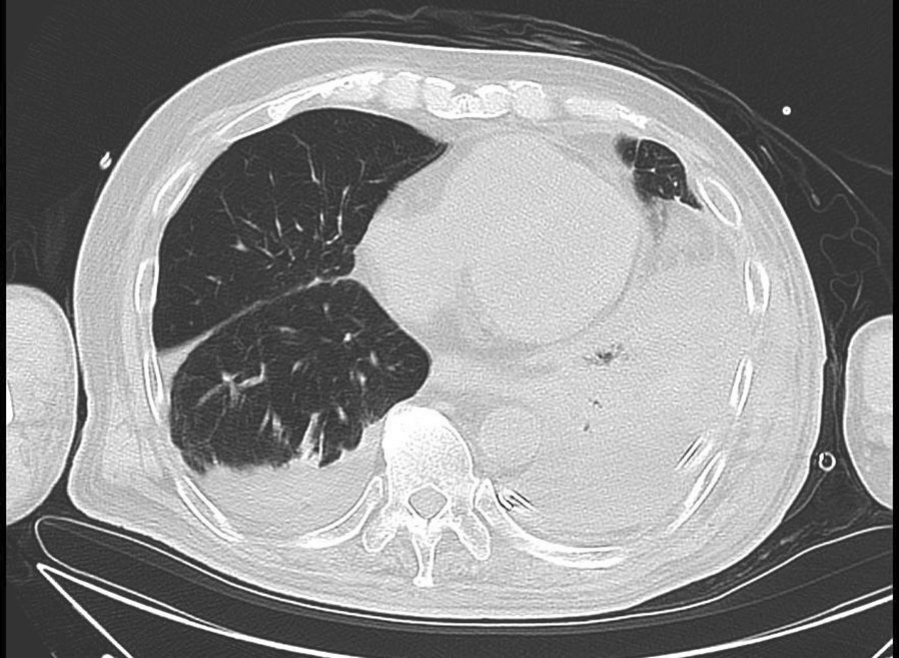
Computed tomography of the chest with pneumohydrothorax and mediastinal air
